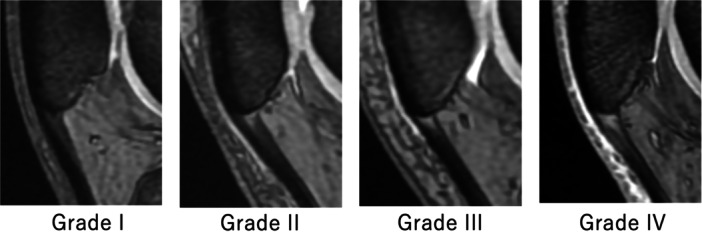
Grading system for tendon MRI signal intensity. Representative sagittal MR images of the quadriceps and patellar tendons at their potential ACL graft harvest sites demonstrate the four‐grade classification system for the signal intensity, based on previous studies. Grade Ⅰ (homogeneous low signal intensity), Grade Ⅱ (high signal intensity regions comprising <50% of the area), Grade Ⅲ (high signal intensity regions comprising ≥50% of the area), and Grade Ⅳ (diffuse high signal intensity). ACL, anterior cruciate ligament; MRI, magnetic resonance imaging.
